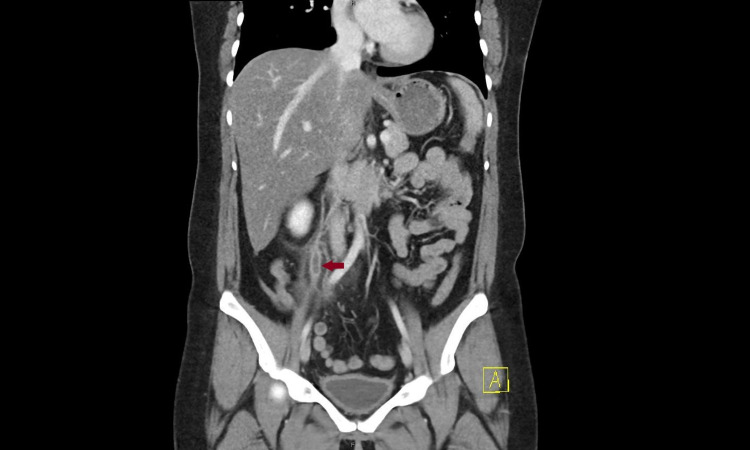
Coronal view of computed tomography scan with contrast of abdomen and pelvis showing right ovarian vein thrombosis (Arrow)
